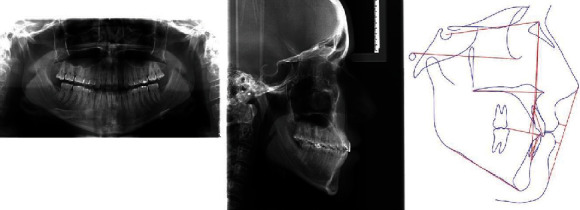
Panoramic and lateral cephalometric radiography after the orthodontic treatment.
